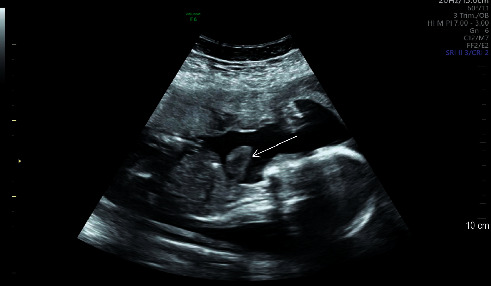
The heart is outside the chest cavity in this ultrasound image of a sagittal view (arrow), making it impossible to assess the nasal bone.
